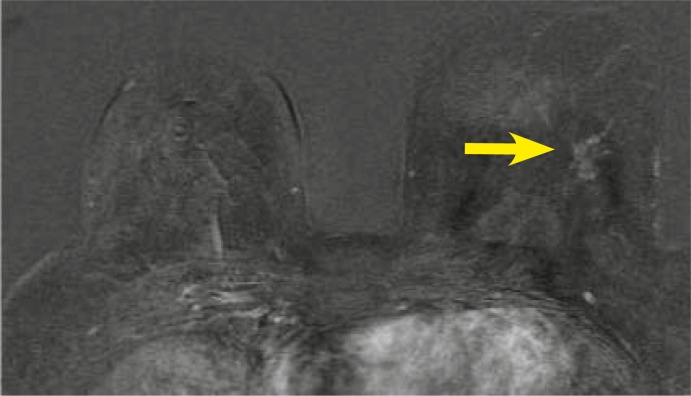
63-year old woman with history of invasive ductal carcinoma in her right breast 8 years ago. Clinical exam revealed enlarged lymph node in the left axilla. Fine needle aspiration biopsy showed metastatic lymph node. Mammographically discrete architectural distortion only in one projection in the left and postoperative changes in the right breast. Ultrasound was not performed at the discretion of the radiologist. Axial T1-weighted subtracted image after Gadolinium injection (2^nd^ minute) shows focal, heterogeneous, asymmetric, non–mass like enhancement in the left breast, the kinetic curve shows fast initial enhancement with post-initial wash out, low signal intensity on T2-weighted images – BI-RADS 4. MRI–guided vacuum-assisted biopsy revealed invasive lobular carcinoma.
